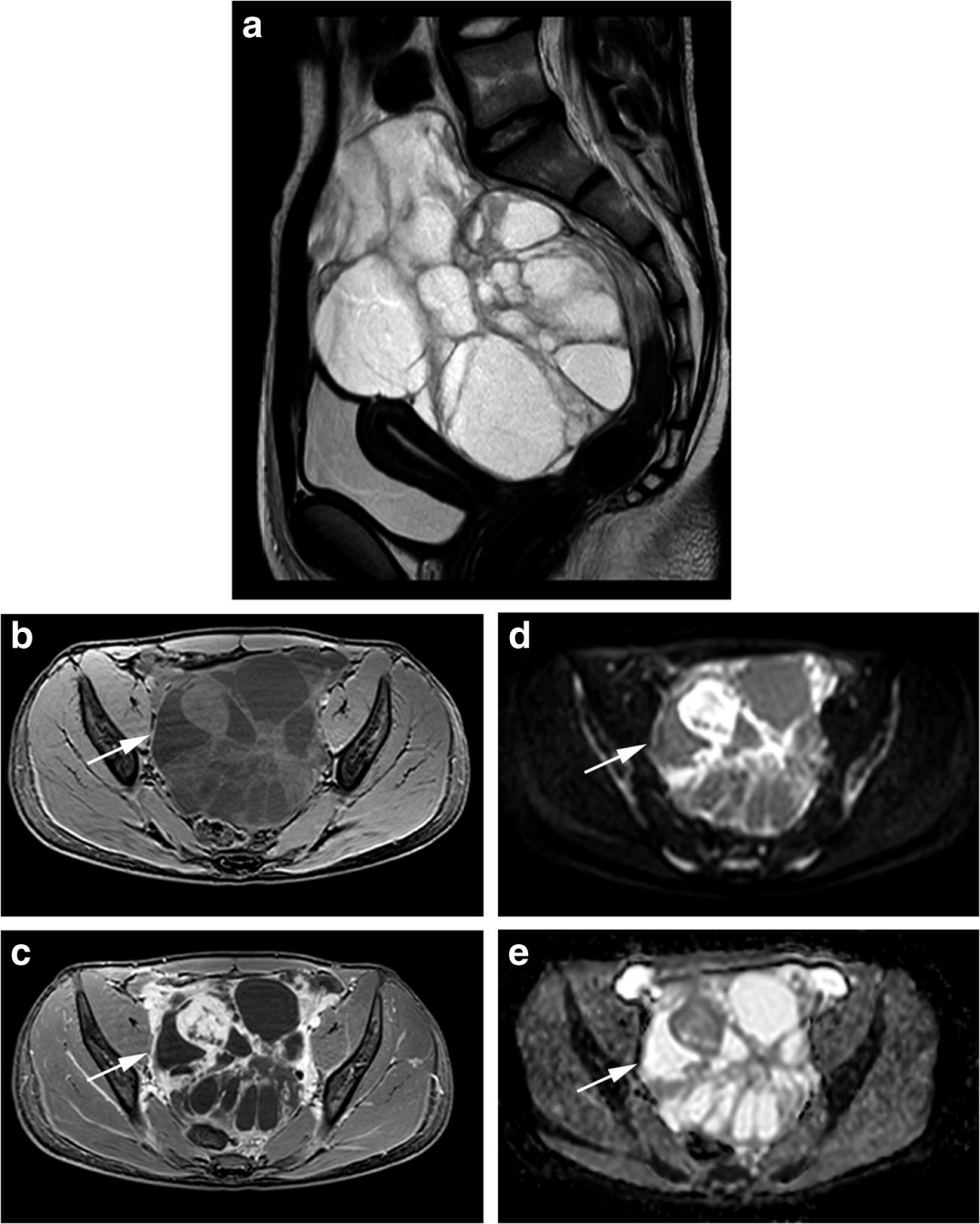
An example of yolk sac tumour of the right ovary in a 16-year-old girl. Sagittal T2-weighted turbo spin echo TSE (a) and T1-weighted gradient echo with fat suppression before and after administration of gadolinium contrast (b, c) show a large cystic solid mass in the lower abdomen. The enhancing parts of the lesion show relative impeded diffusion (arrow) at axial DWI (b1000 and ADC map; d,e)
